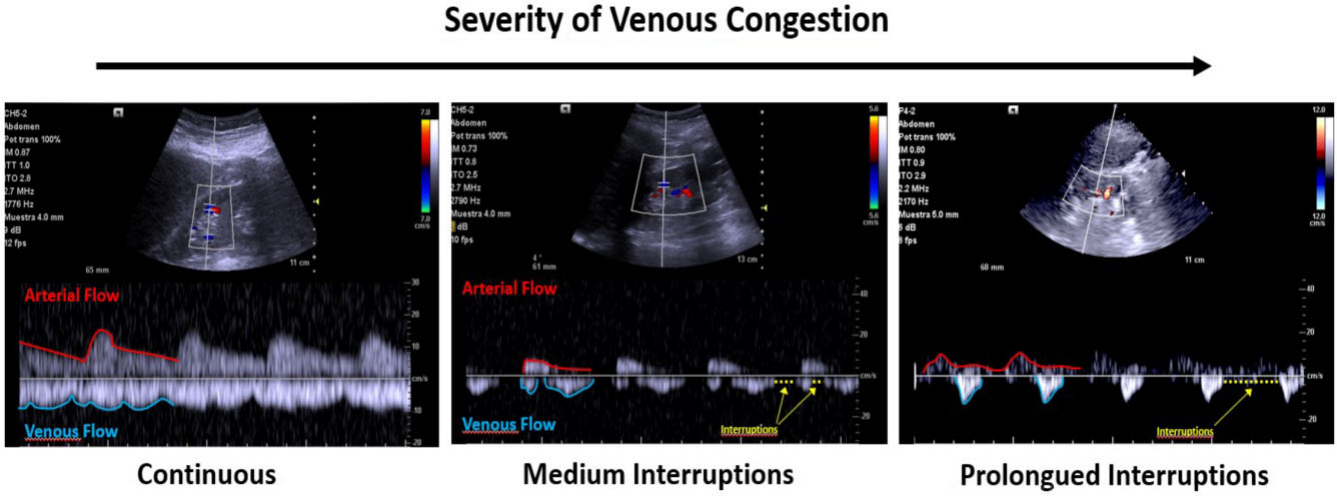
Assessment of renal venous flow in the presence of congestion. Under normal conditions, intrarenal venous flow is continuous and can be observed by pulsed Doppler below baseline (blue line). Renal venous flow is interrupted (yellow dots) and becomes biphasic or monophasic as systemic congestion increases. Note that low resistance arterial flow appears above baseline (red line).
